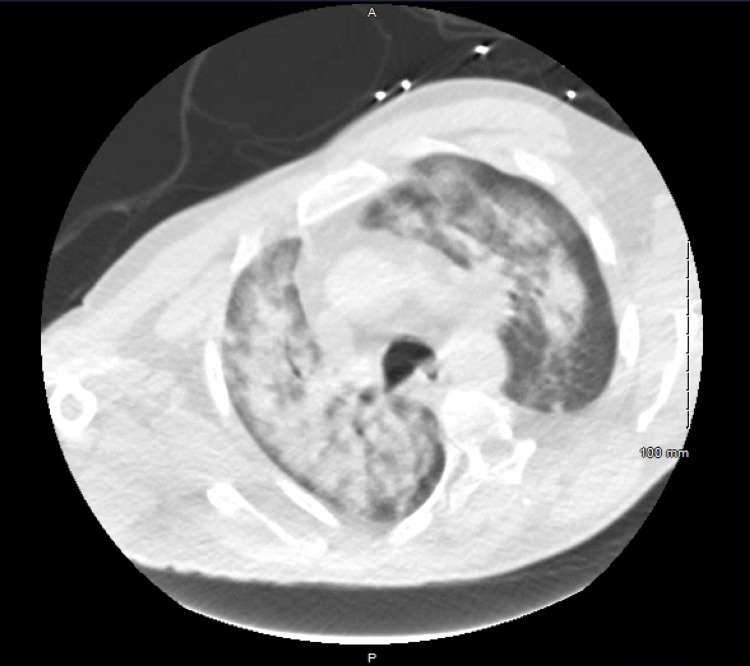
Chest computed tomography with intravenous contrast demonstrating bilateral ground-glass opacities.
